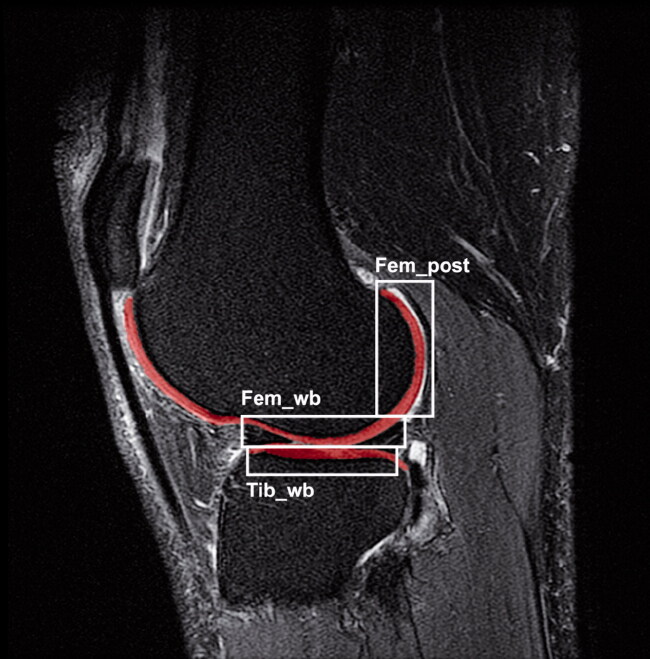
Cartilage segmentation on a T2-weighted image of the lateral compartment. Red area displays the femoral and tibial cartilage; white boxes represent the ROIs. Abbreviations: Fem_wb: weight-bearing femoral condyle; Fem_post: posterior femoral condyle; Tib_wb: weight-bearing tibial plateau.
